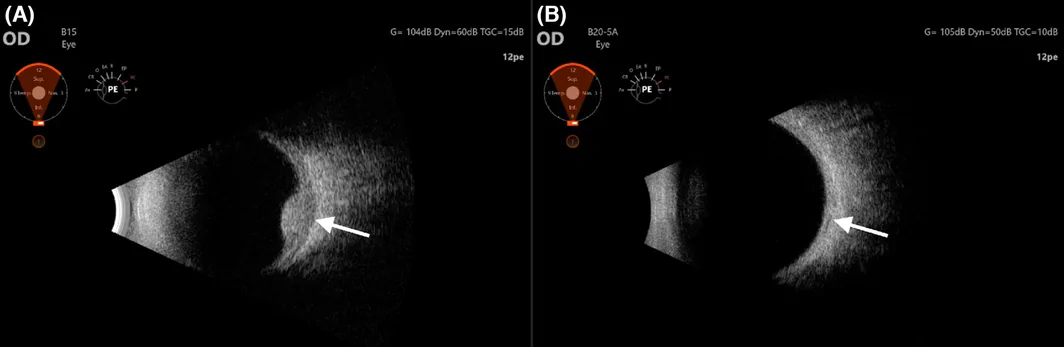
Right eye ocular ultrasound B‐scan. (A) Dome‐shaped choroidal elevated lesion (white arrow). (B) Repeat B‐scan 1 month post initiation of osimertinib shows resolution of previously noted choroidal mass. [Color figure can be viewed at wileyonlinelibrary.com]
